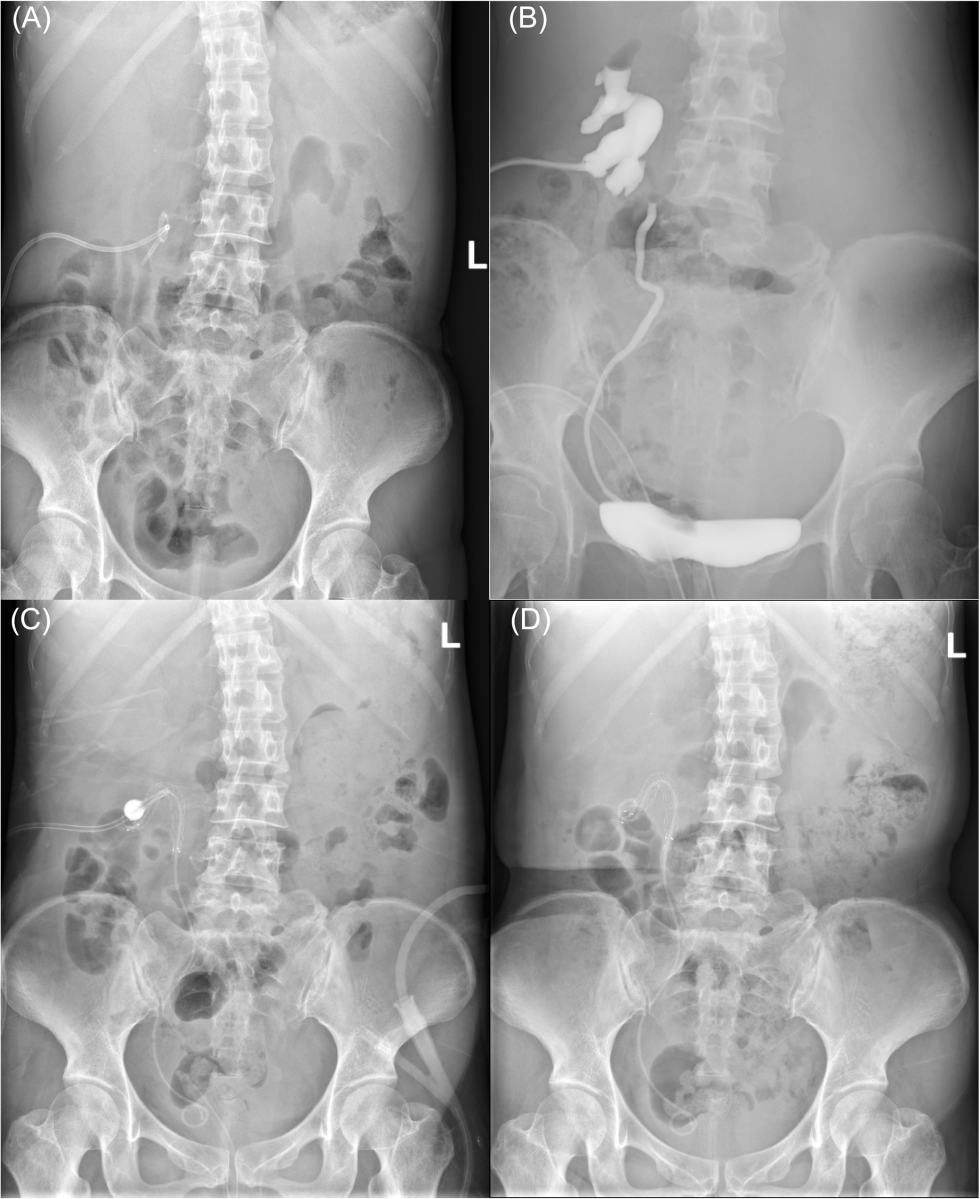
Imagings of the patient with right ruptured ureter before and after Allium stent insertion. (A) kidney, ureter and bladder (KUB) radiography showing the broken double‐J stent in right kidney, nephrostomy tube for urine drainage; (B) Antegrade and retrograde pyelography demonstrating the defect of contrast medium in right‐upper ureter; (C) KUB radiography showing the exact position of Allium stent bridging right ruptured ureter 2 weeks after surgery; (D) KUB demonstrating the exact position of Allium stent bridging right ruptured ureter 3 weeks after surgery without nephrostomy tube.
